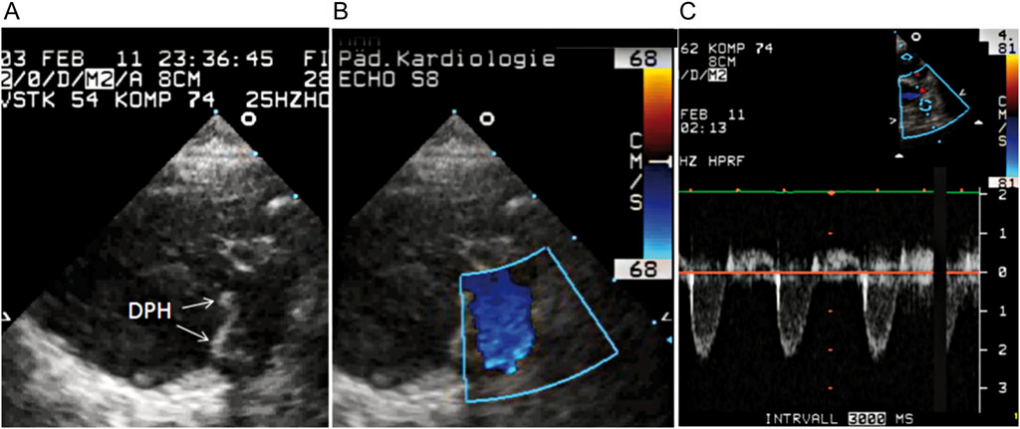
Postoperative echocardiography in a 16-week old girl, who died 3 months after DPH implantation due to sepsis. (A) 2-dimensional echocardiography in the short-axis view along the right ventricular outflow tract and DPH; (B) Colour-Doppler image at DPH in systole; (C) pulse-wave Doppler signal at DPH level showing laminar flow and mild regurgitation.
